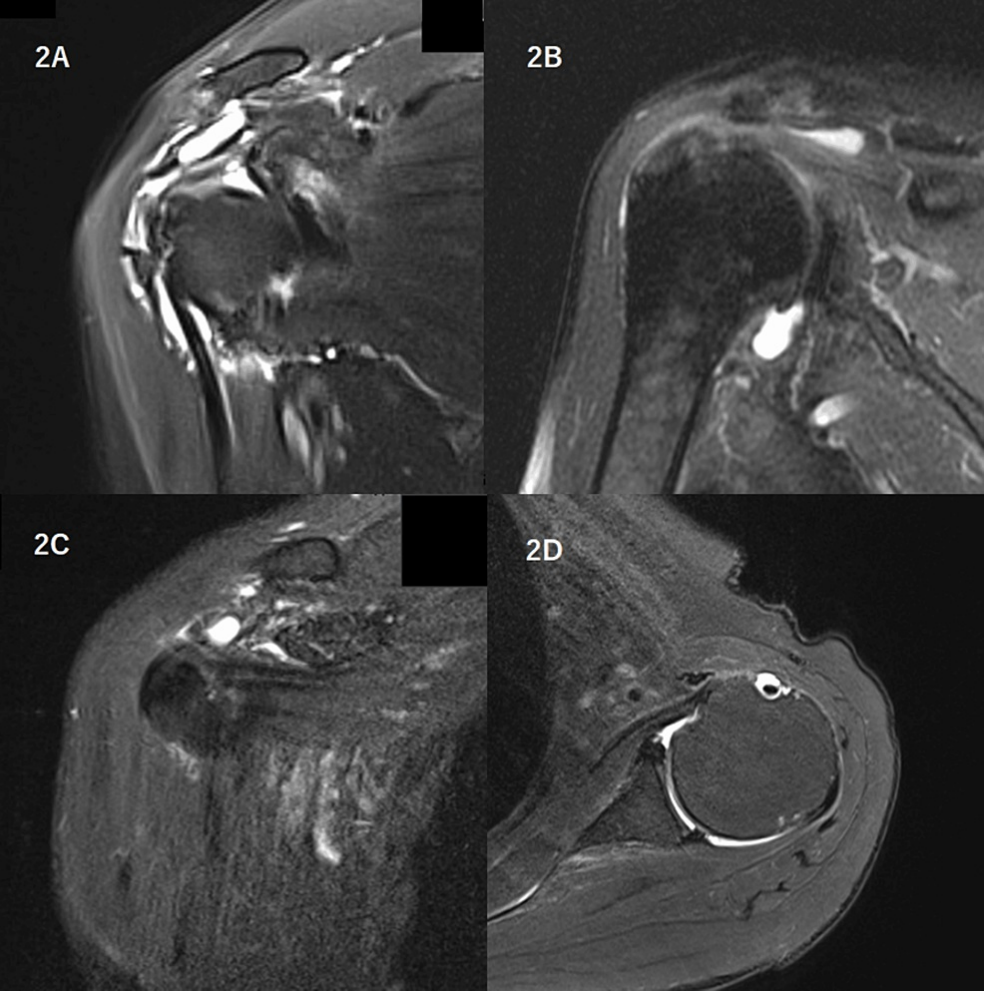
Magnetic resonance imaging. (A) Case 1: inflamed tissues around right shoulder, (B) Case 2: fluid in subacromial bursa, (C) Case 4: fluid in bursa at ventral side of acromioclavicular joint, (D) Case 5: fluid in bursa of long head of biceps brachii. MRI was not obtained for Case 3.
